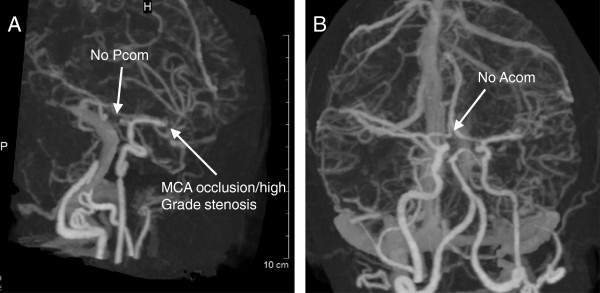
Subtracted 3D CT angiogram following CVA. Oblique view showing high-grade stenosis of left MCA with M2 M3 branches opacified by retrograde flow through leptomeningeal collaterals (A). Neither posterior communicating artery (A) nor anterior communicating artery (B) visible upon imaging in oblique and AP views, respectively.
